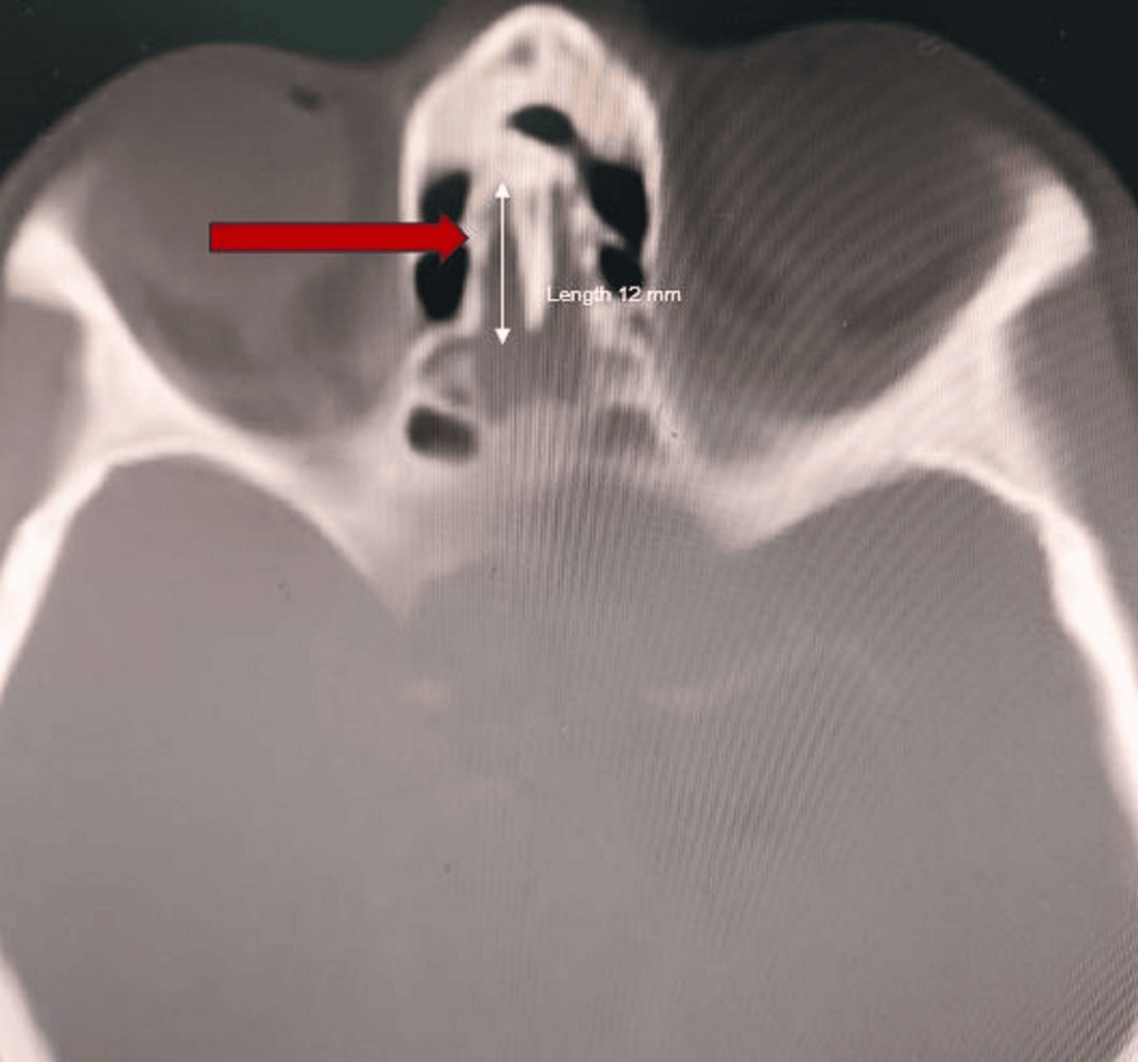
Axial section of CT scan paranasal sinuses showing right sided type 2 Yenigun
